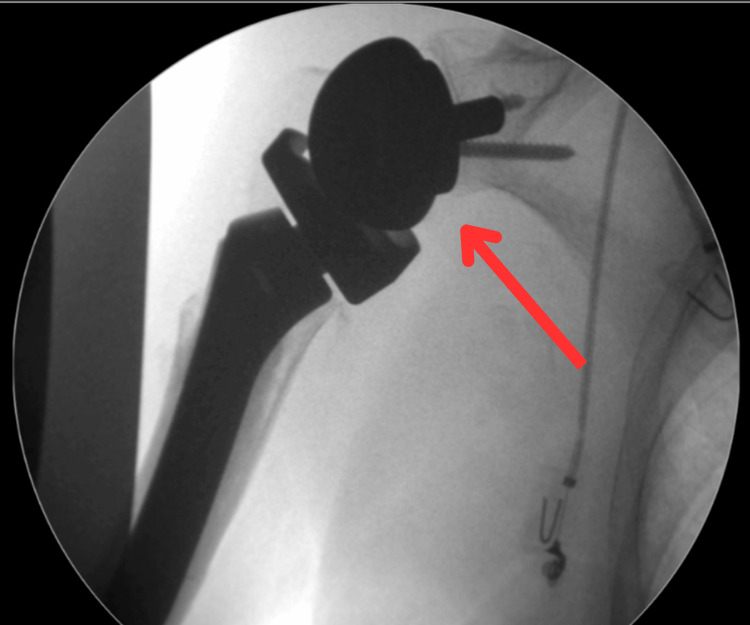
Post-MUA radiograph MUA: manipulation under anaesthesia
